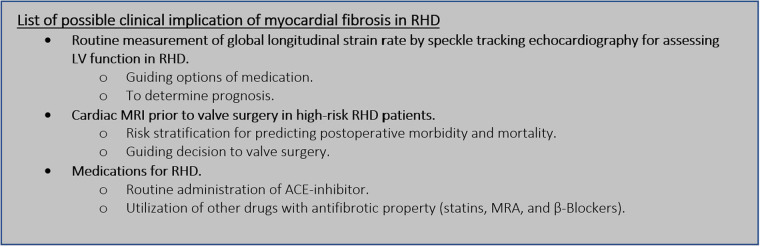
Future perspective of myocardial fibrosis in RHD. LV, left ventricle; RHD, rheumatic heart disease; MRI, magnetic resonance imaging; ACE, angiotensin converting enzyme; MRA, mineralocorticoid receptor antagonist.
